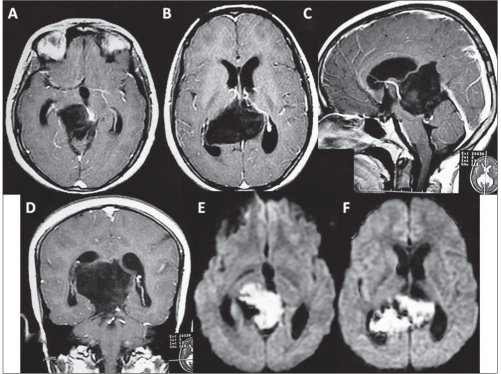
Patient 2: A, B: This 16-year-old girl presented with symptoms of raised intracranial pressure, Perinaud’s syndrome and gait ataxia. Her axial contrast T1-weighted images showed the nonenhancing irregular lesion in the posterior third ventricular region and the ambient and quadrigeminal cisterns. There is mild hydrocephalus with dilated anterior third ventricle and bilateral lateral ventricles. C: Sagittal, and D: Coronal contrast T1 images showing the vertical extent of the lesion along the brain stem and its extension into the supratentorial compartment after occupying the tentorial incisural space. The superior vermis of the cerebellum appears flattened by the lesion. E, F: Diffusion-weighted axial images showing the restriction of diffusion in the lesion.
